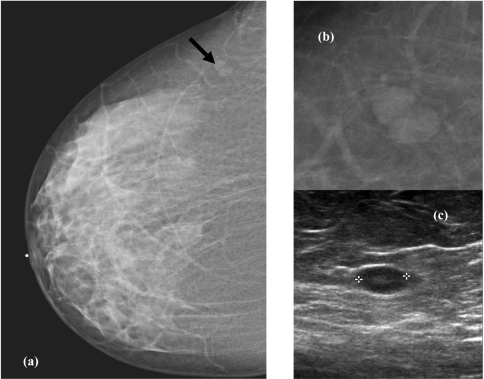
(a) Nodule at right outer quadrant on right CC view (black arrow). (b) Magnification of the nodule shows a rim of lucent halo suggestive of a benign nodule. (c) Ultrasound confirms a benign intramammary lymphnode with a central fatty hilum.
